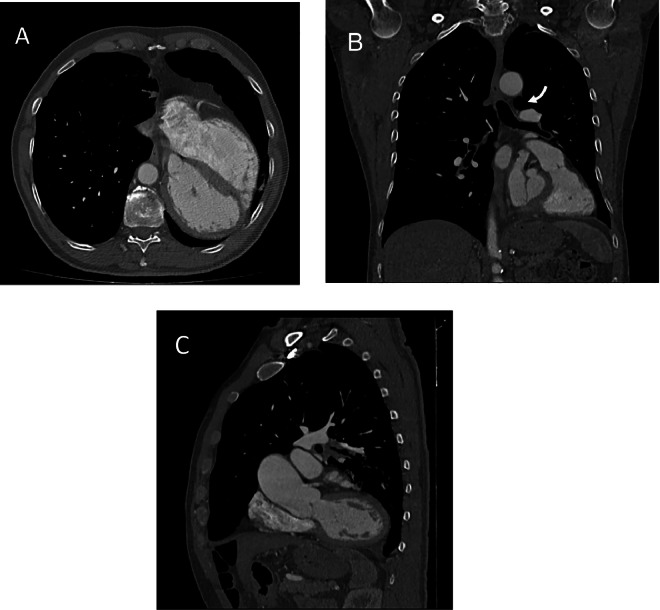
Cardiac computed tomography imaging demonstrating complete pericardial agenesis (A-C): *(A) Transaxial section; noticeable levorotation and levoposition of the heart (B) Coronal section; a conspicuous notch between the aorta and the pulmonary artery (arrow) (C) Sagittal section; posterior position of the apex*.
